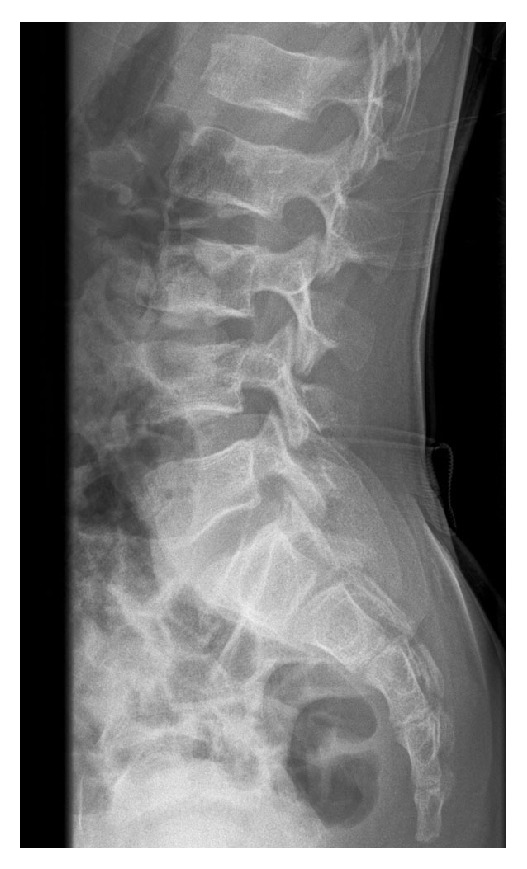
Lateral radiograph of the lumbar spine shows normal L3-L4 disk and normal endplates of L3 and L4.
